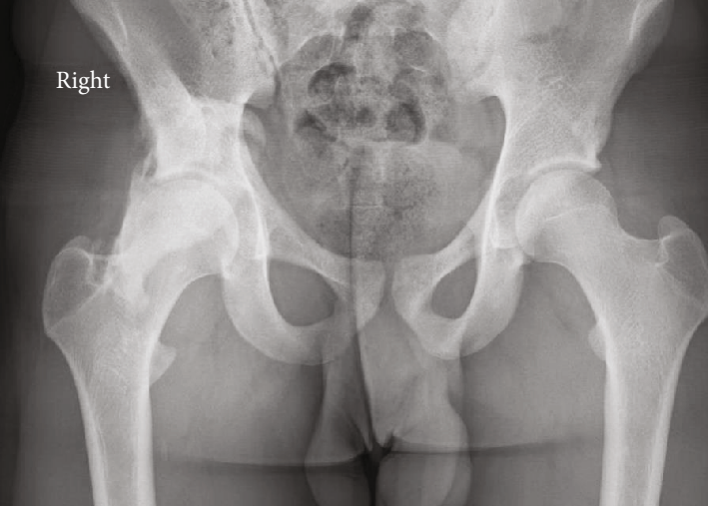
AP X-ray of the pelvis demonstrating an avulsion fracture of the right AIIS with large amount of bony callus.
